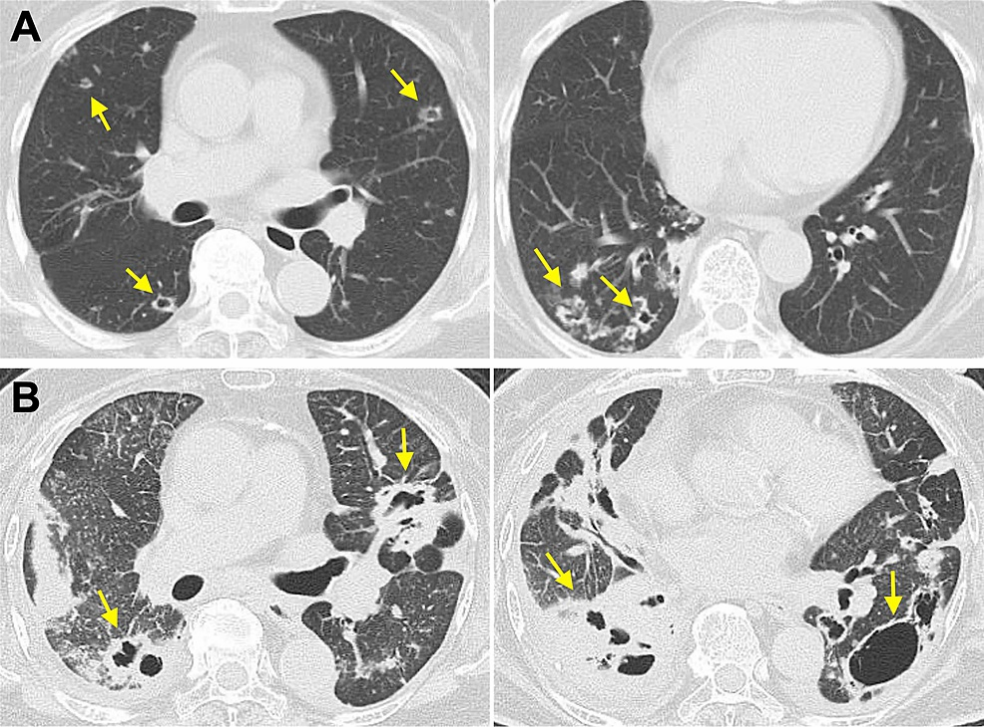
Chest CT findings. (A) Multiple pulmonary nodules with cavities (arrows). (B) The progression of multiple pulmonary nodules with cavities (arrows), thickening of interlobular septa and bronchovascular bundles, and bilateral pleural effusions, 13 months later. CT, computed tomography
